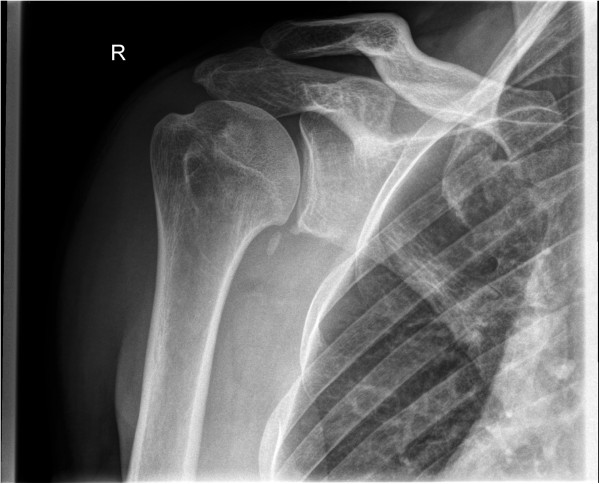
Preoperative X-rays in anteroposterior projection.
